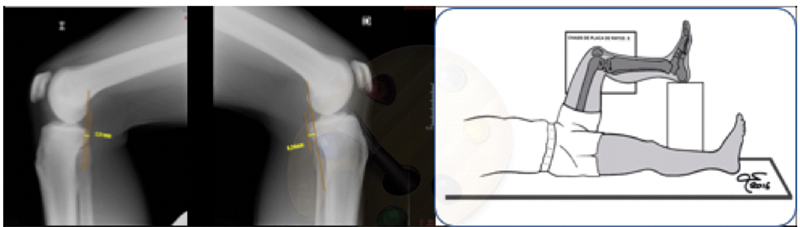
Stress X-rays (gravitational) showing a partial tear of isolated posterior cruciate ligament injury (Courtesy of Dr. Xavier Cuscó).
